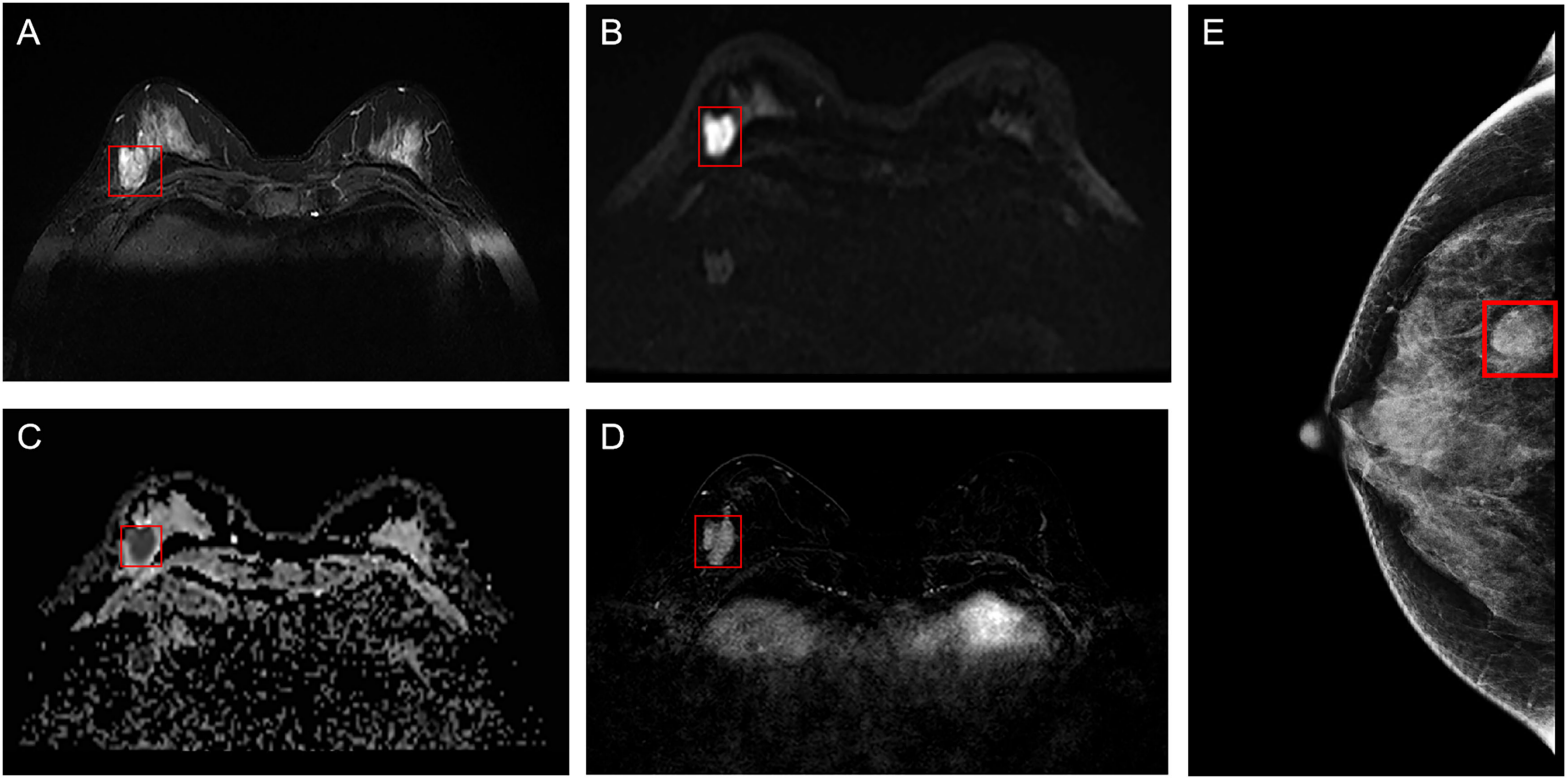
Example of ROI segmentation from raw MRI and MG images of breast cancer. (A) T2WI of the breast; (B) DWI of the breast; (C) ADC of the breast; (D) Period with the most significant enhancement in DCE-MRI of the breast; (E) MG of the breast. ROI, region of interest; MG, mammography; T2WI, T2-weighted imaging; DWI, diffusion weighting imaging; ADC, Apparent dispersion coefficient; DCE-MRI, dynamic contrast-enhanced magnetic resonance imaging.
